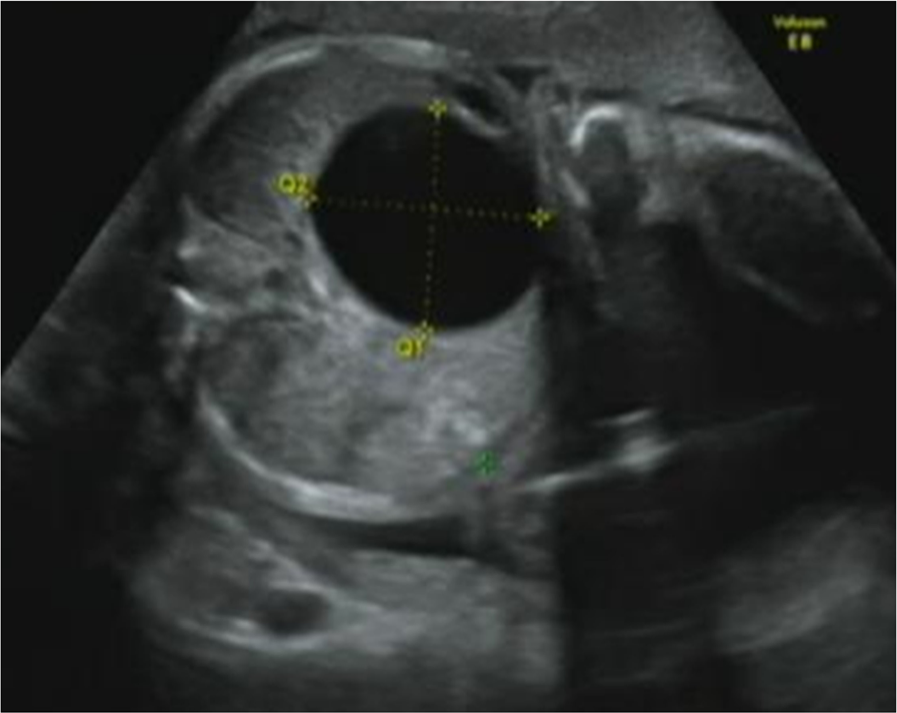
Antenatal ultrasonographic findings at 16 weeks gestation. A cystic lesion, 37 × 40 mm in size, was observed in the hepatic hilum
